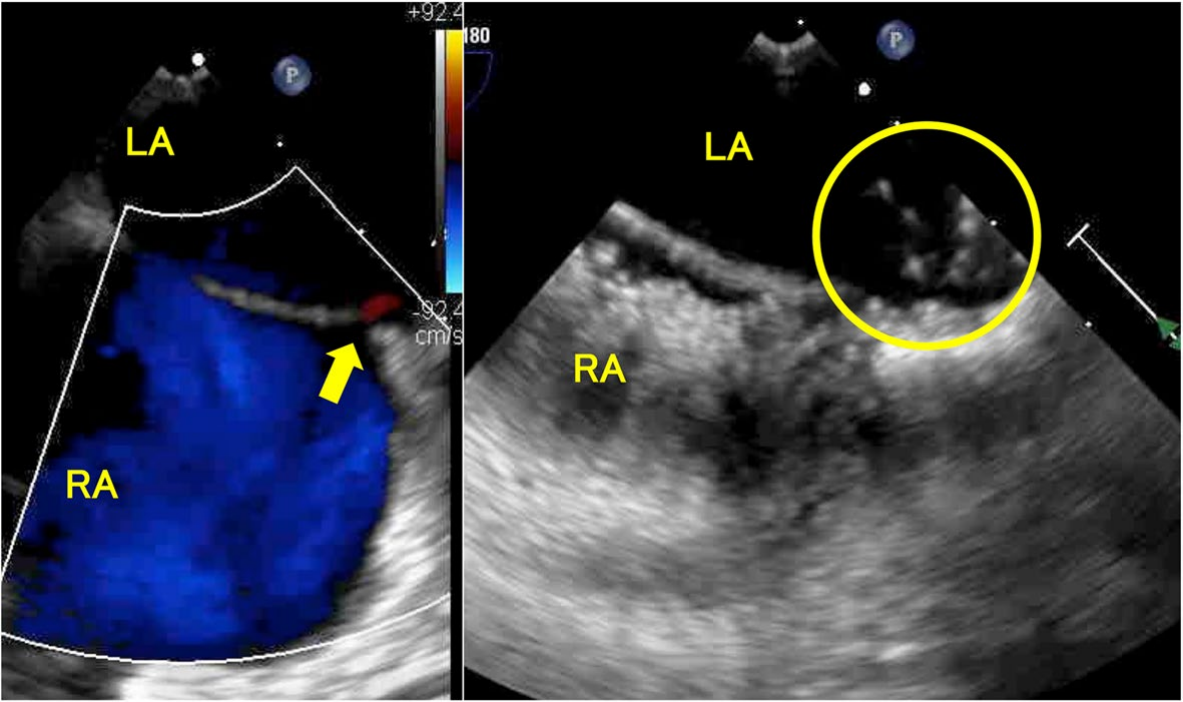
Transesophageal echocardiography findings. The left figure showed a 1.5 mm slit-like defect, patent foramen ovale in the fossa ovalis, and right-to-left shunt blood flow (arrow) observed during the Valsalva maneuver. The right figure showed the microbubbles (circle) from the right to the left atrium during the Valsalva maneuver. RA, right atrium; LA, left atrium
